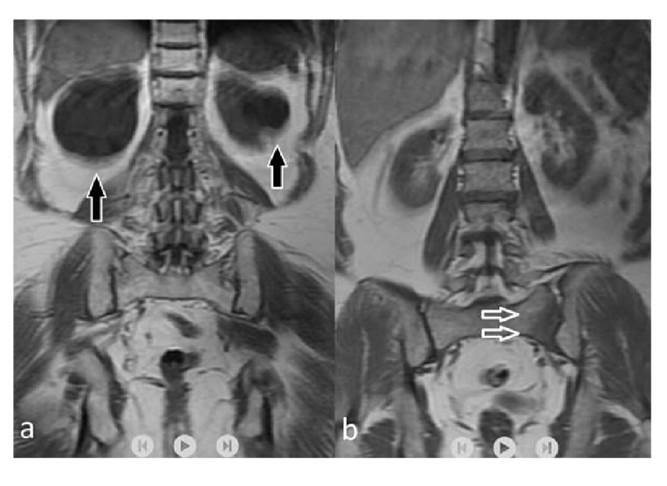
Coronal T1-weighted images show hypointense cysts (arrows) located in both kidneys (a). On the coronal-T 1 weighted sequence (b), a unilateral sacral hypointensity at the level of sacroiliac joints was detected (arrow); this finding is consistent with unilateral sacroiliitis and it was not detectable on conventional MR sequences.
